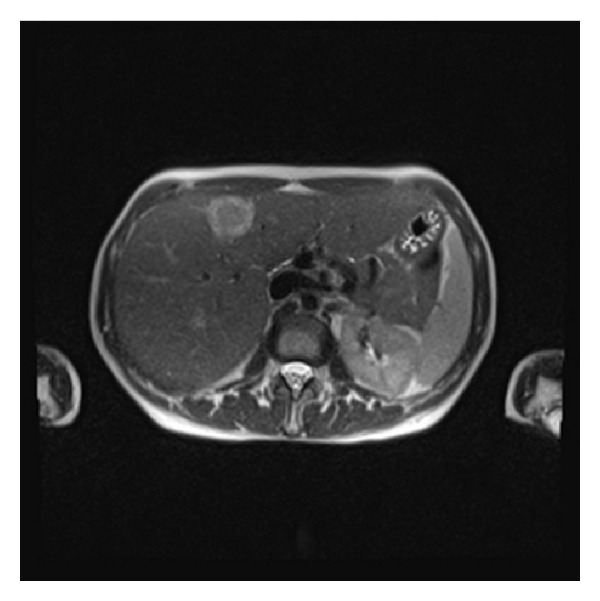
T2 hyperintense liver lesion on MRI.
